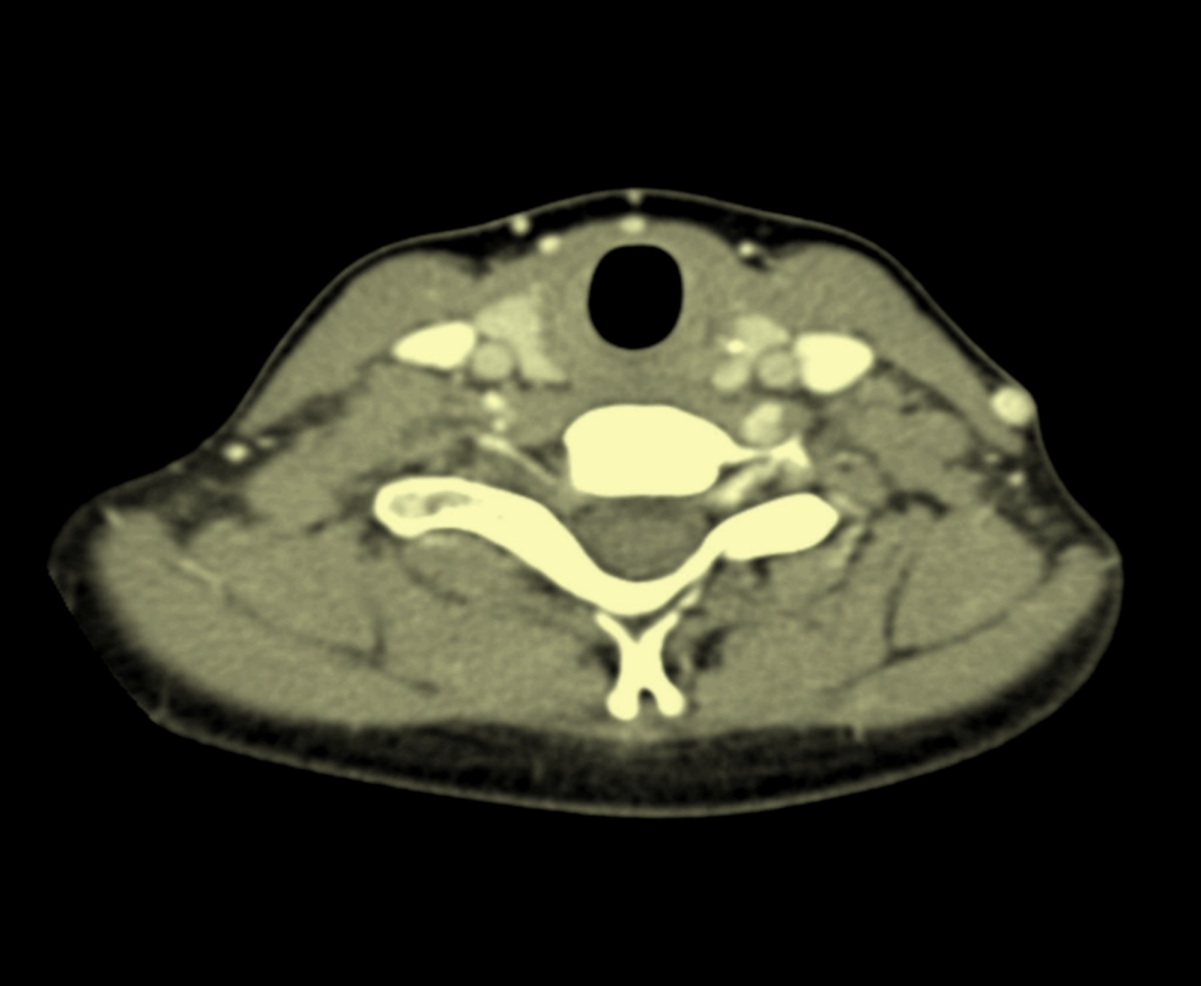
Contrast-enhanced computed tomography (CT) of the neck showing the absence of cervical metastases
